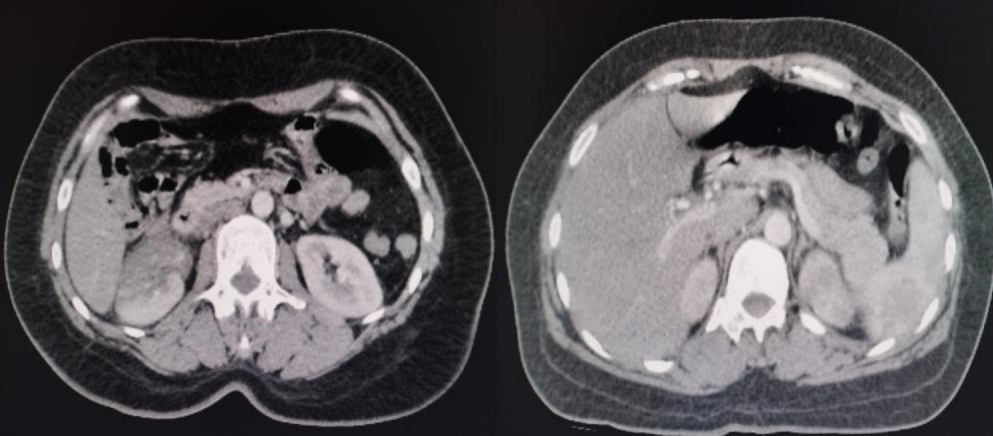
Computed tomography image showing right renal infarct (image in the left) and splenic infarct (image in the right).
